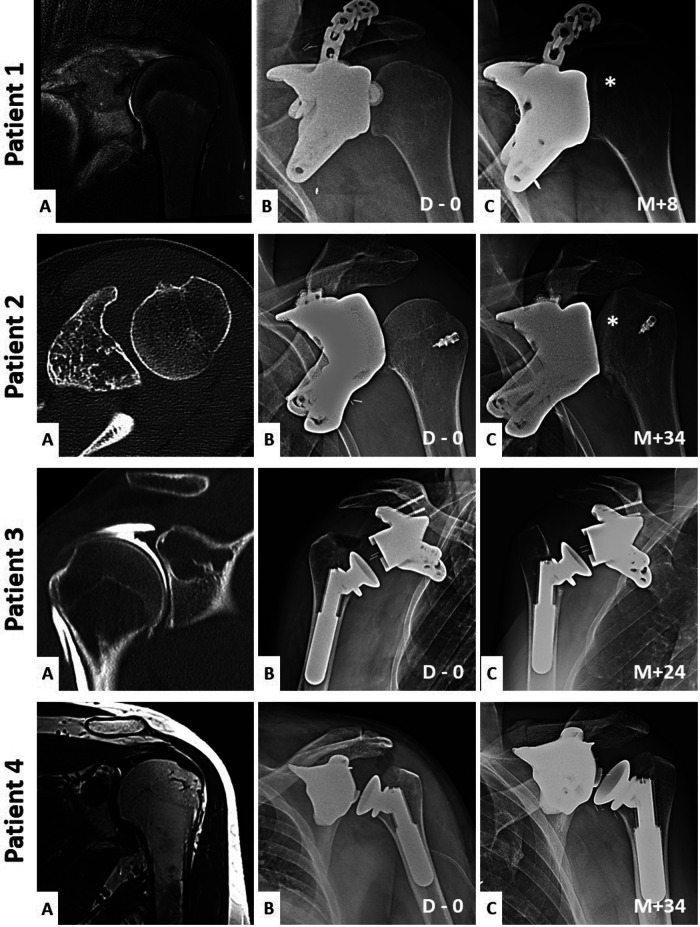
Preoperative and short and long-term imaging of the patient's shoulder. Patient (1-A) is a coronal T2 MRI sequence. Patient (2-A) is a coronal T1 MRI sequence. Patient (3-A) is a coronal injected arthro-CT acquisition. Patient (4-A) is an axial CT-scan acquisition. Each (A) images show the initial pathology. Each (B) images show the immediate postoperative x-rays of the shoulder's reconstructions (D = days). Each (C) images show the long-term postoperative x-rays of the reconstruction's evolutions (M = months). Asterisks show the humeral head deformations when a hemiarthroplasty is used.
